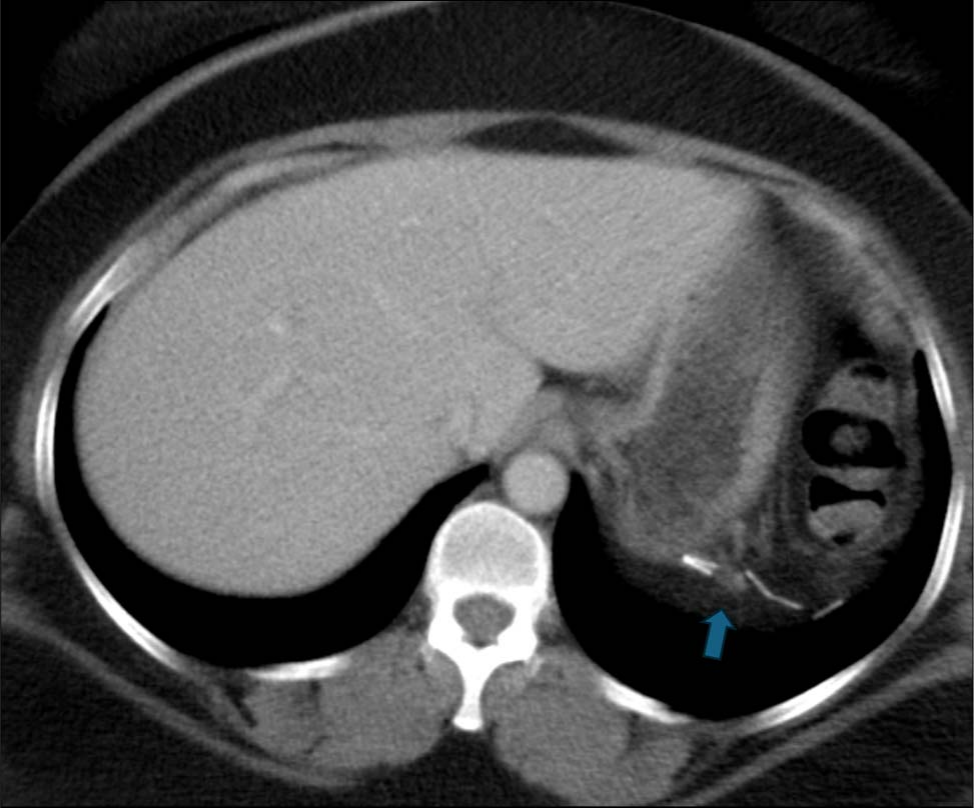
Axial computed tomography scan image of the abdomen and pelvis with intravenous contrast only shows splenectomy clips in the left upper quadrant abutting the stomach (blue arrow).
